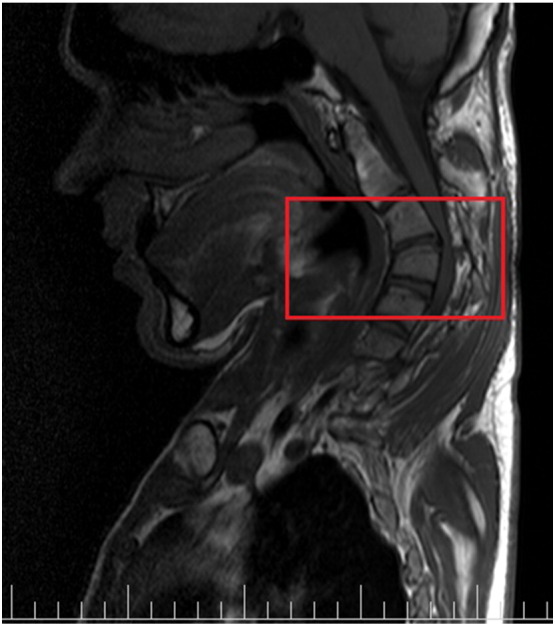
C3-C4 spinal cord compression in cervical MRI.
